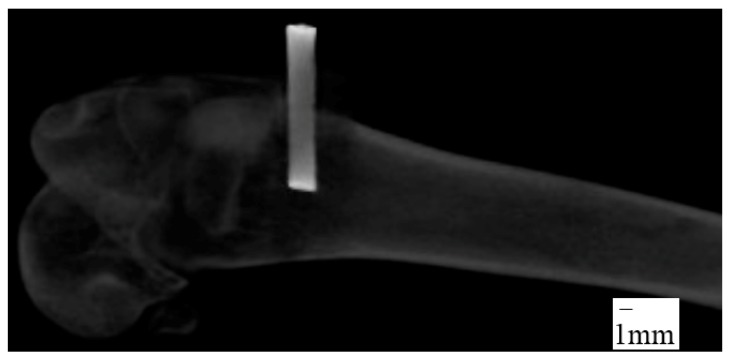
X-ray image of femur after surgery.
